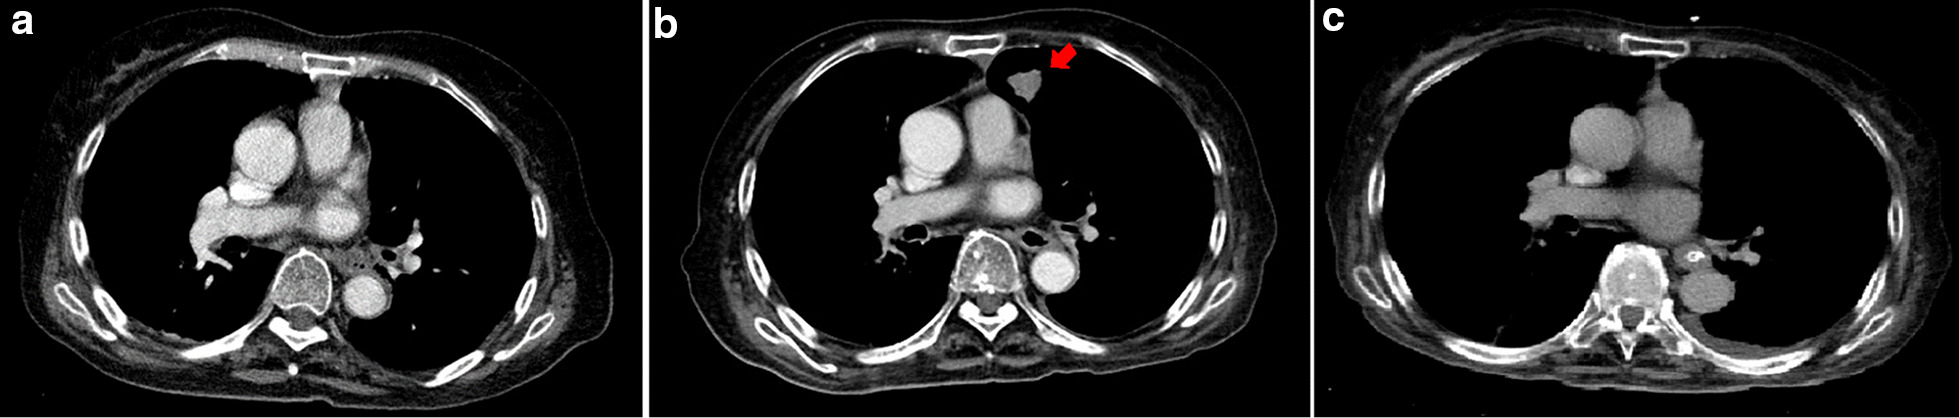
CT of the chest of nearly the same sections, at different times. CT performed a at admission in the emergency department revealed no active lung lesions. b At 3 months after admission, a newly developed solid nodule in the upper lobe of the left lung (red arrow) was observed. c At 3 months of fluconazole therapy, complete clearance of the lesion is observed
